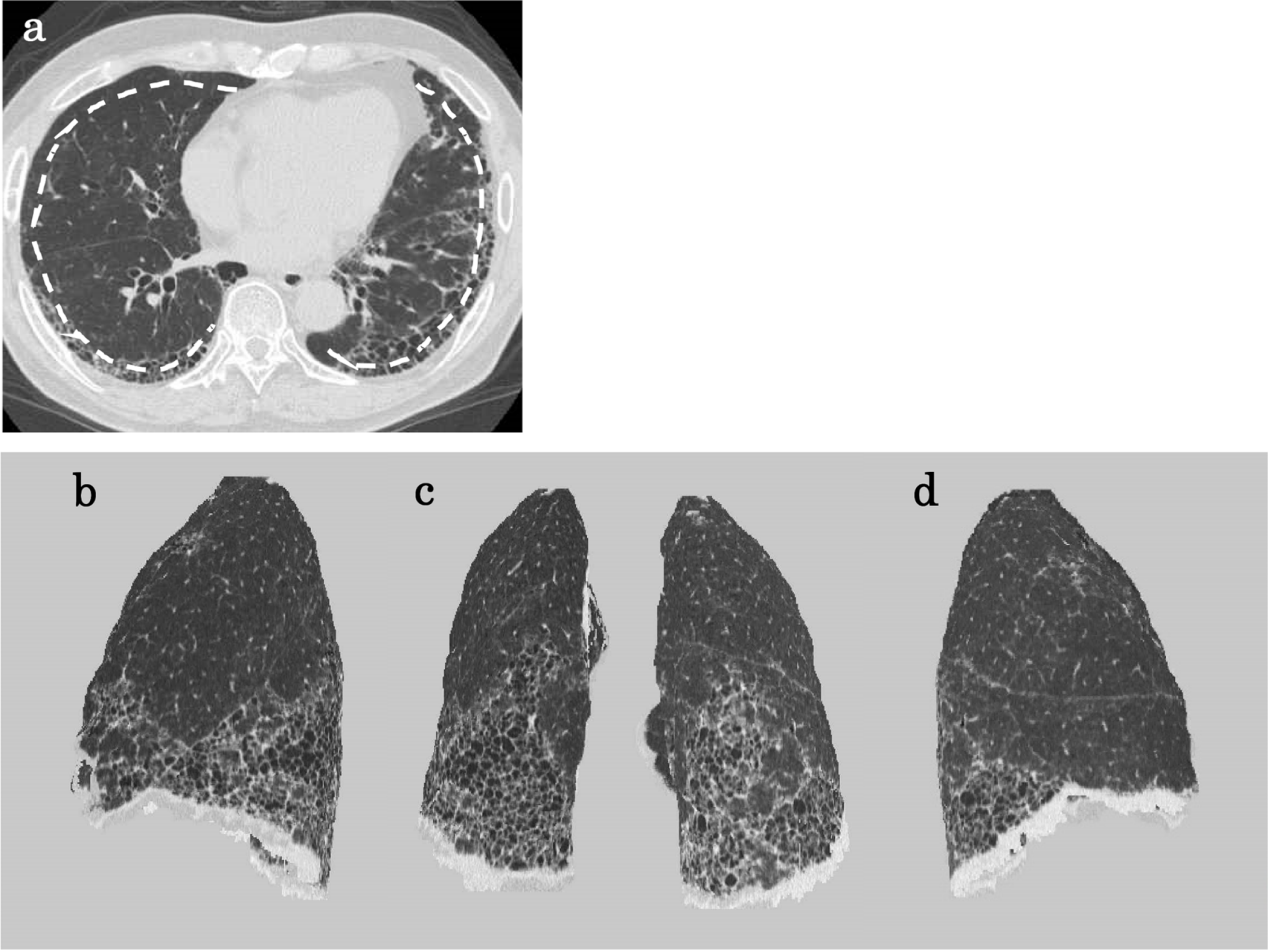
A patient with clinical and radiological typical usual interstitial pneumonia. A patient with clinical and radiological typical usual interstitial pneumonia. (a) Axial HRCT image and dashed line indicating 1-cm depth from the chest wall; multiple-view 3D-cHRCT images showing the 3D distribution of IP infiltrates; (b) left lateral view, (c) posterior view, (d) right lateral view.
